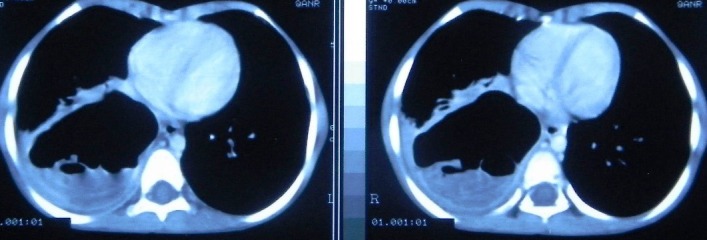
Figure 2: CT thorax showing an 8.5 x 7.5 x 6.5 cm air-filled cavitary lesion with detached dependent wavy membrane in the right lower zone of lung consistent with hydatid cyst.
